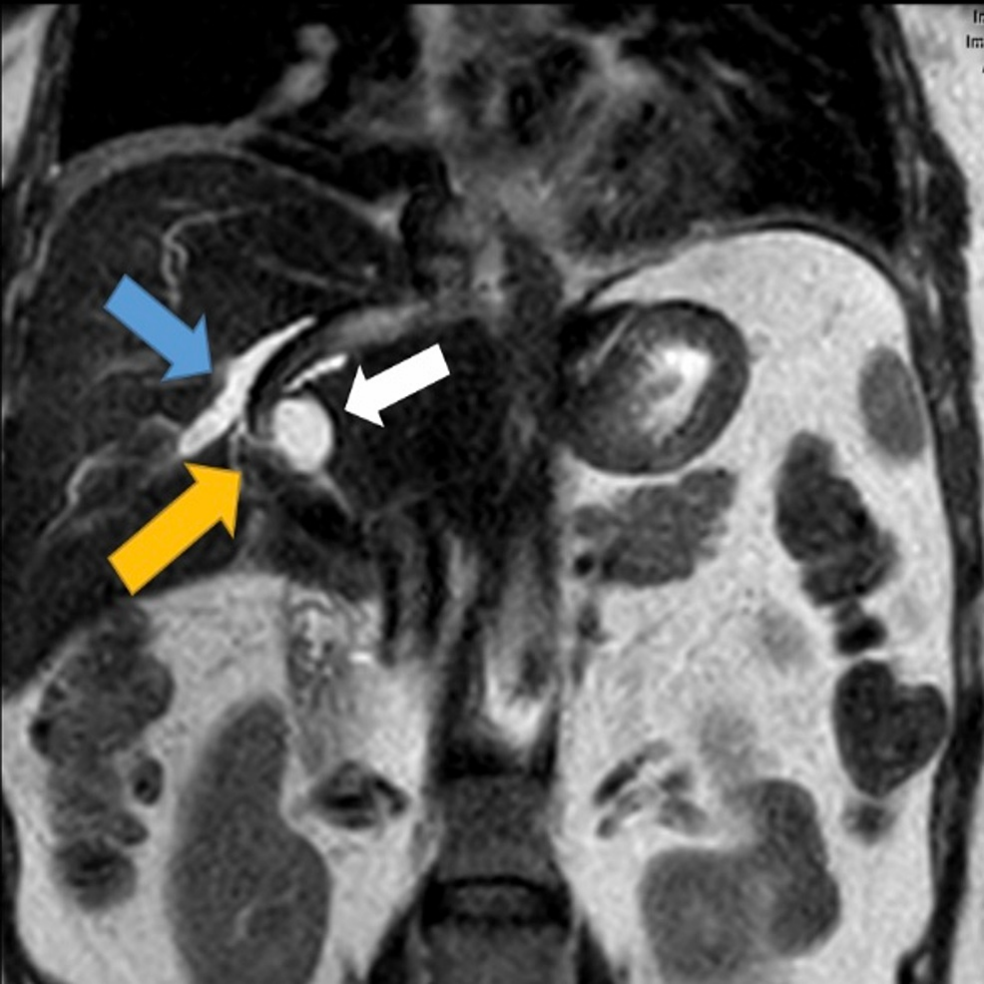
MRI abdomen, coronal T2-weighted image, shows a new intrahepatic biloma (white arrow) abutting a portion of the TIPS (yellow arrow) with upstream intrahepatic biliary ductal dilatation (blue arrow). TIPS: transjugular intrahepatic portosystemic shunt
